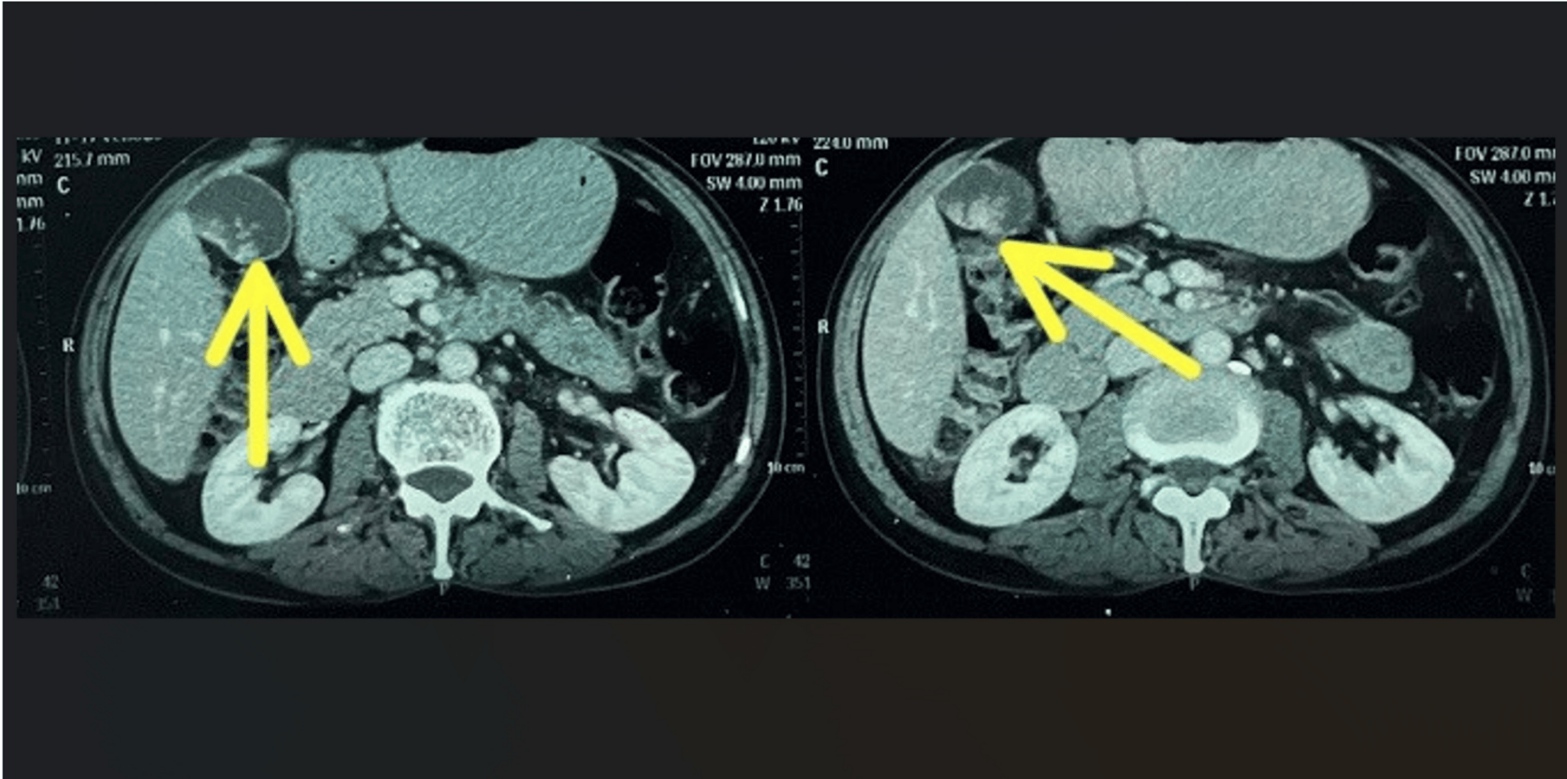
CT scan demonstrating a mass in the gallbladder during the portal venous phase (yellow arrow). CT: computed tomography
